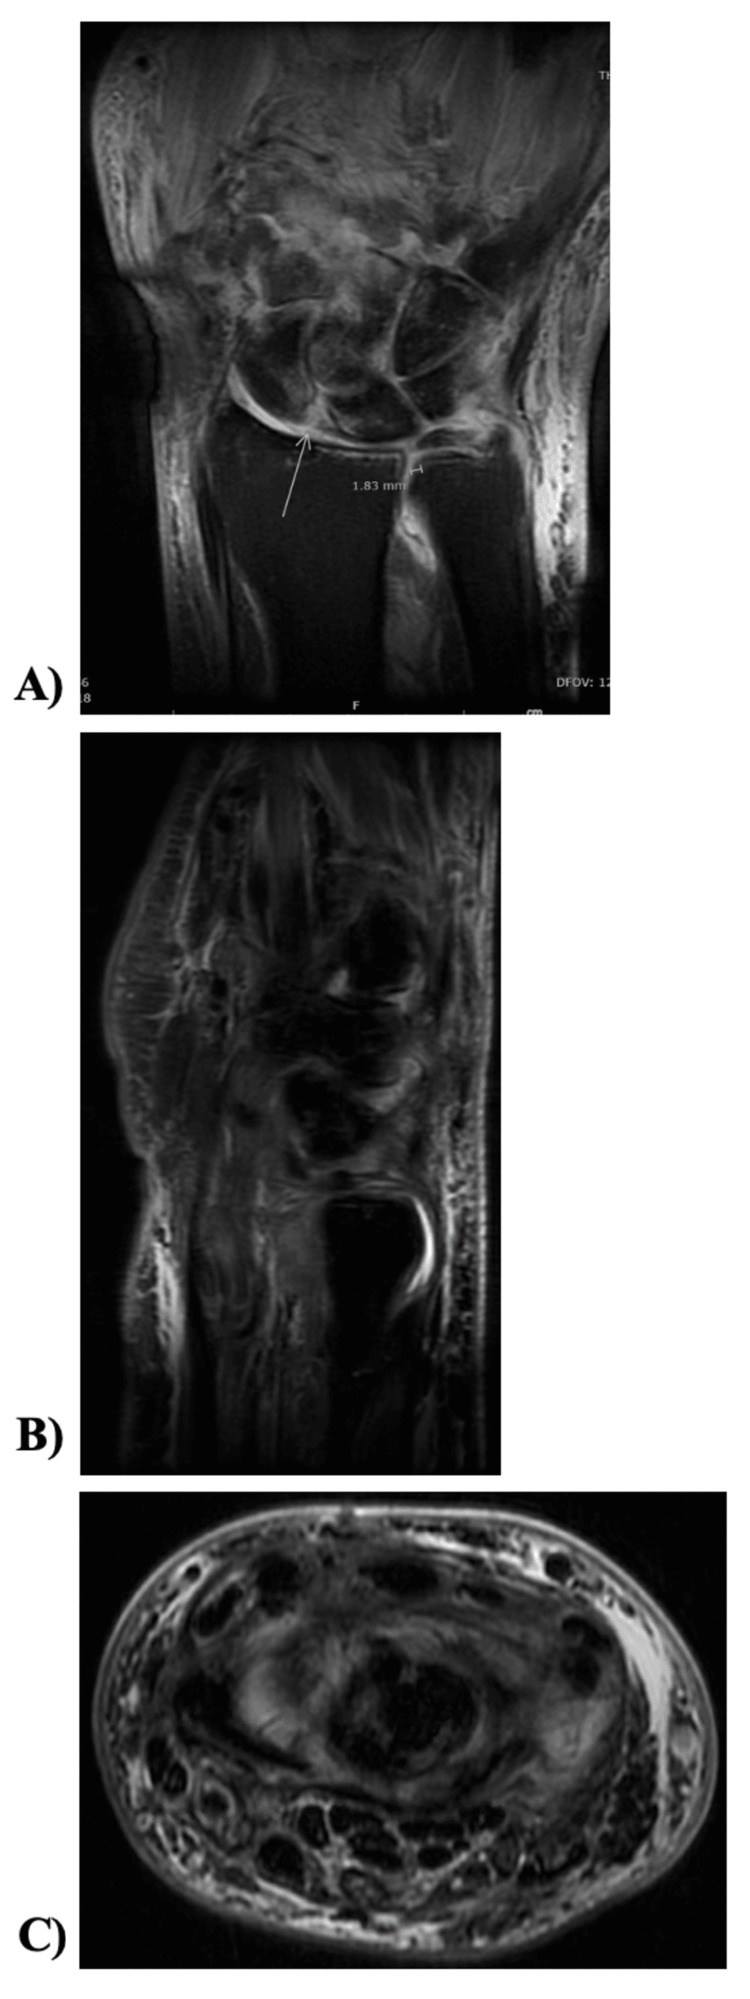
Repeat left wrist MRI after six weeks of symptoms. MRI of left wrist, six weeks after irrigation and debridement for septic arthritis. (A) Coronal, (B) sagittal, and (C) axial views showing moderate enhancing synovitis and capsulitis of the midcarpal, radiocarpal (green arrow), and distal radioulnar joints and worsening bone marrow signals of the proximal second and third metacarpals, captitate, and trapezoid with appearance of osteomyelitis.
